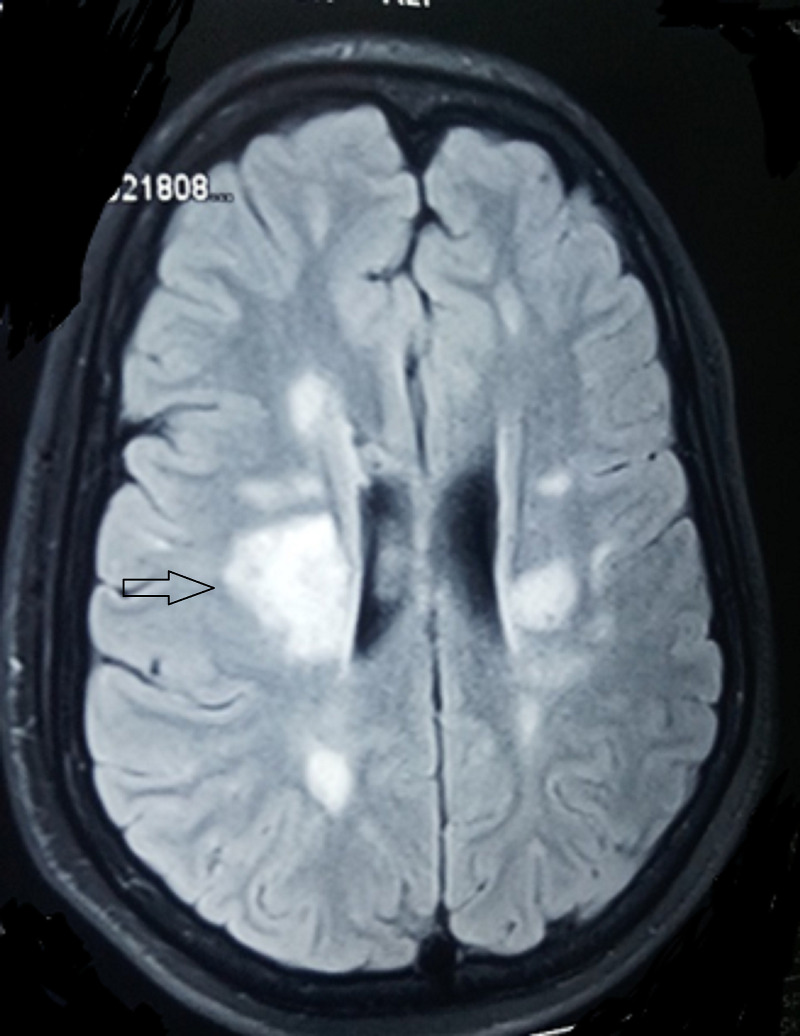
Hyperintense white matter lesions (arrow) on T2-weighted MRI brain supportive of multiple sclerosis diagnosis MRI: magnetic resonance imaging
